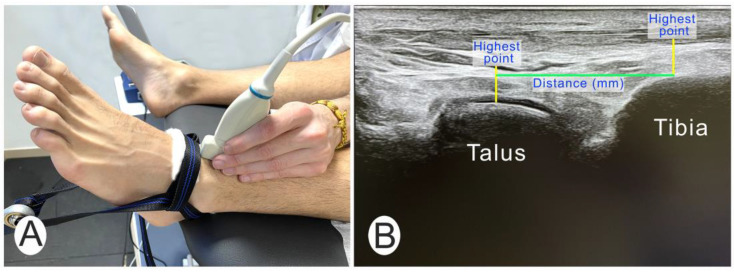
(A). Transducer position at anterior part of ankle joint. (B): Ultrasound image of tibia and talus at rest as baseline.
